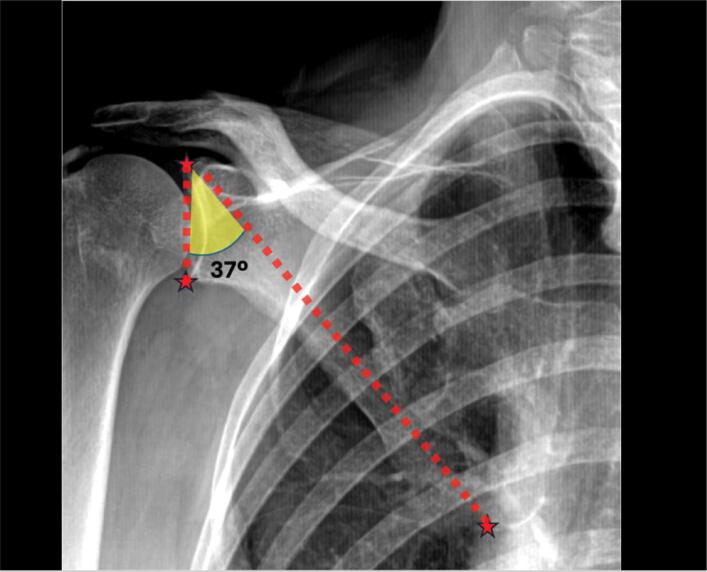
Measurement of the glenopolar angle in a true AP shoulder radiograph.
